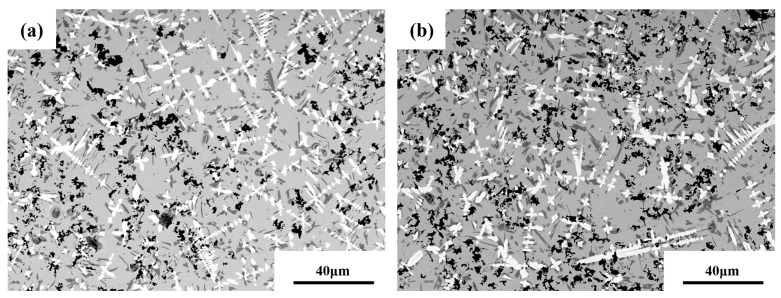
Microstructure at the coating top (a) with and (b) without ultrasonic vibrations (UP = 800 W, F = 32 kHz).
